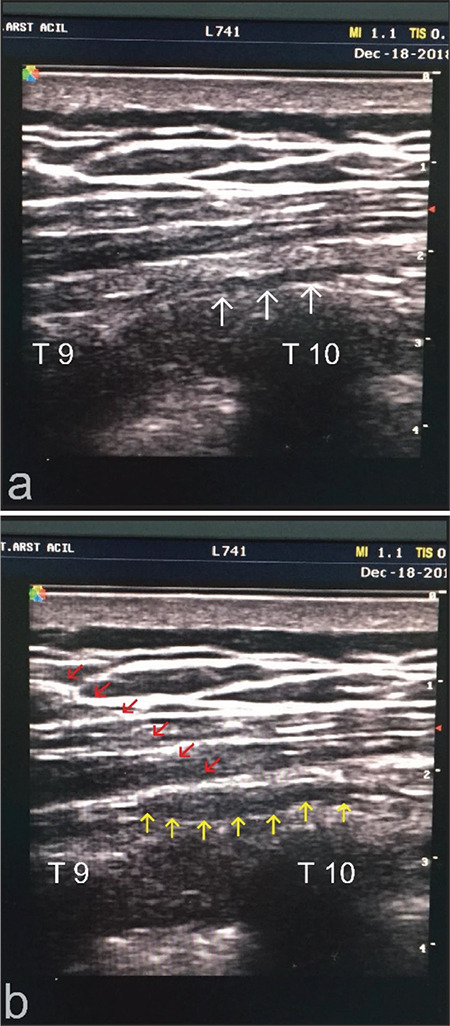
Ultrasound image of ESPB. a. Before the application of local anaesthetic. b. After the application of local anaesthetic through the catheter. T9: Costotransverse joint of T9 vertebrae, T10: Costotransverse joint of T10 vertebrae, white arrows: the lower fascia of erector spinae muscle group, red arrows: shadow of the erector spinae block catheter, yellow arrows: local anaesthetic filled area. ESPB, erector spinae plane block.
